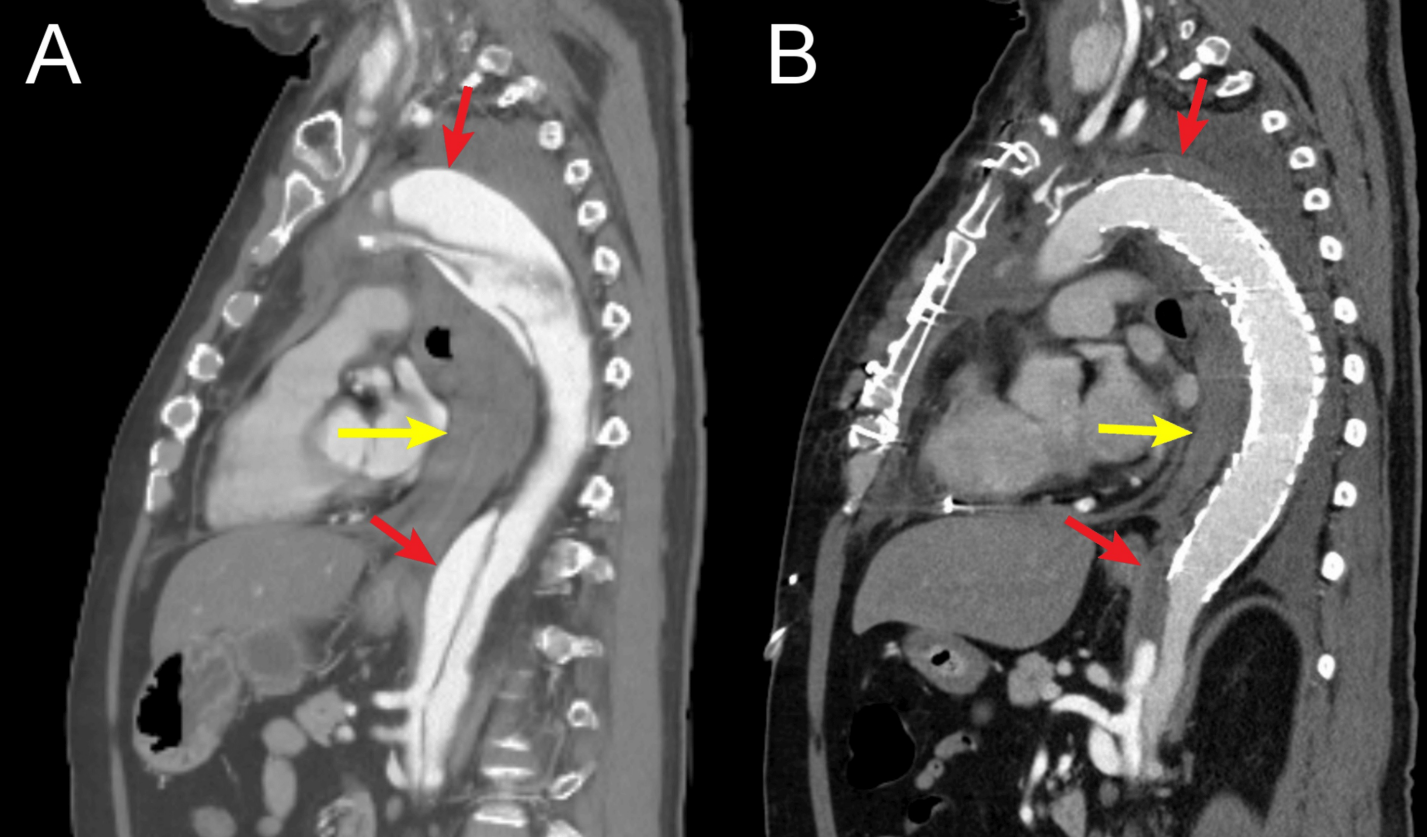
Preoperative and postoperative aortic contrast-enhanced computed tomography (A) Preoperative CECT showing a ruptured dissecting aneurysm of the distal aortic arch. The false lumen (indicated by red arrow) and a periaortic hematoma (indicated by yellow arrow) are clearly visualized. (B) Postoperative CECT demonstrating complete thrombosis of the false lumen along the entire length of the frozen elephant trunk (FET) deployment (red arrow) and a marked reduction in the periaortic hematoma (yellow arrow).
